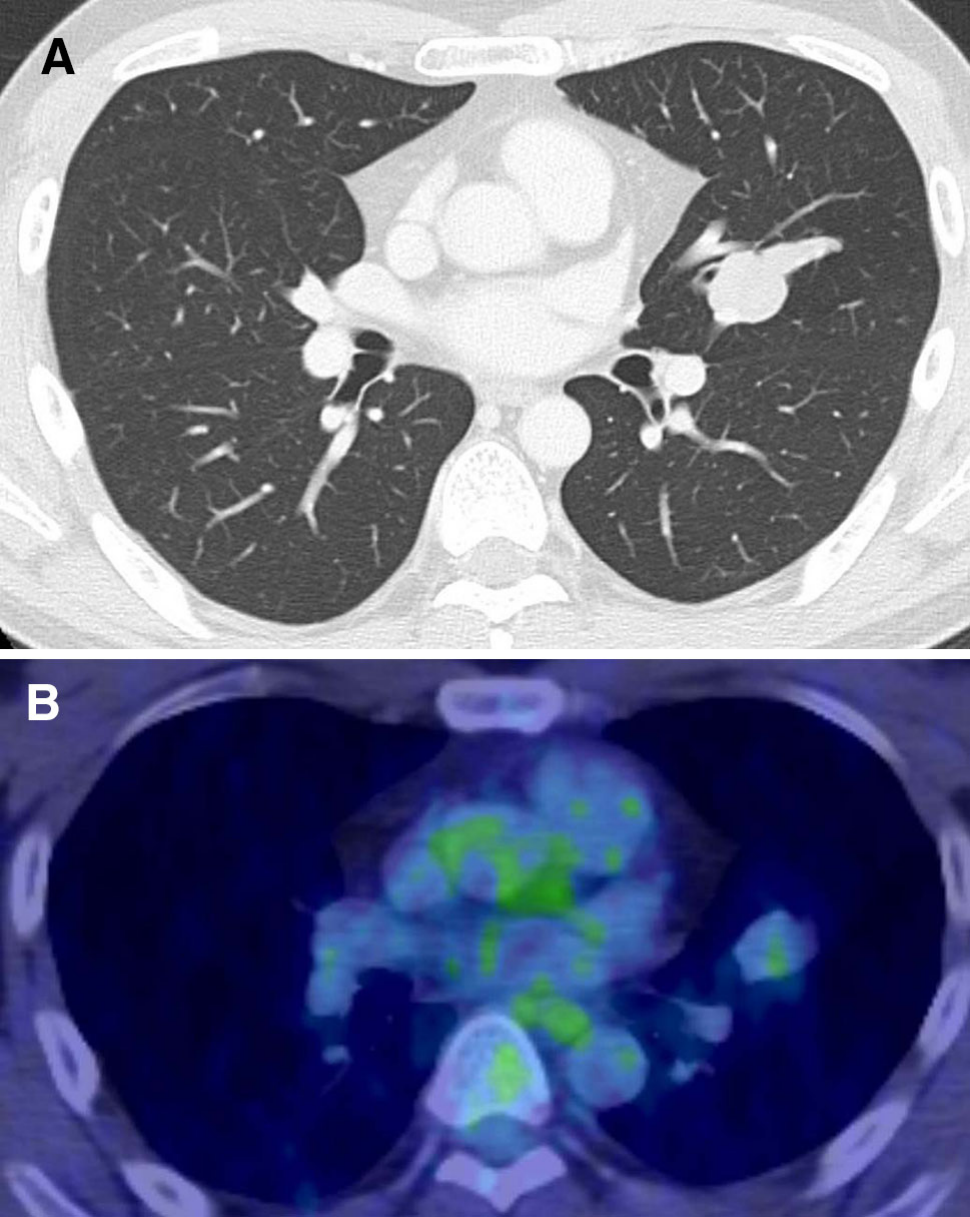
a Computed tomography shows a mass with well-defined margins in the lingular segment of the left upper lobe. b 18-Fluorodeoxy-glucose (FDG) positron-emission tomography (PET) showed no accumulation in the tumor
